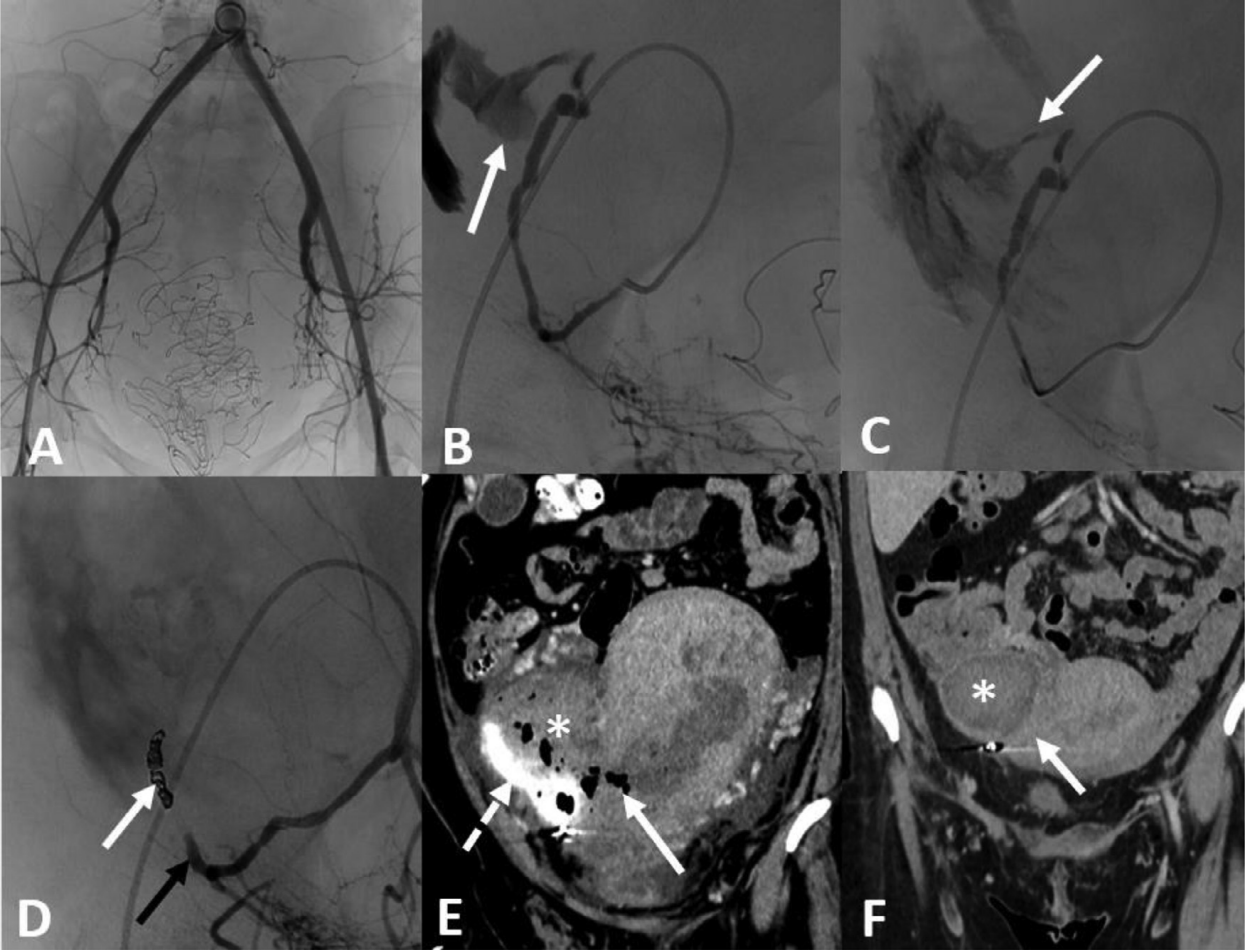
(A) Aortography after placement of 5F right femoral catheter, showing no active bleeding. (B) The selective introduction of a catheter into the right uterine artery shows active bleeding into the peritoneal cavity. (C) Angiography after injection of resorbable gelatin sponge shows slight but persistent, active bleeding. (D) After placement of the microcoils (arrow), angiography shows satisfactory occlusion of the uterine artery without active bleeding. (E) Enhanced CT scan in coronal section the day after embolization shows a gravid uterus associated with a right latero-uterine hematoma (star) with retention of contrast medium (dotted arrow), associated with a defect in enhancement and air the right uterine wall (arrow). (F) CT scan in coronal section performed 1 month after AUE shows a reduction in the size of the hematoma (star) and a restitution ad-integrum of the uterine wall.
